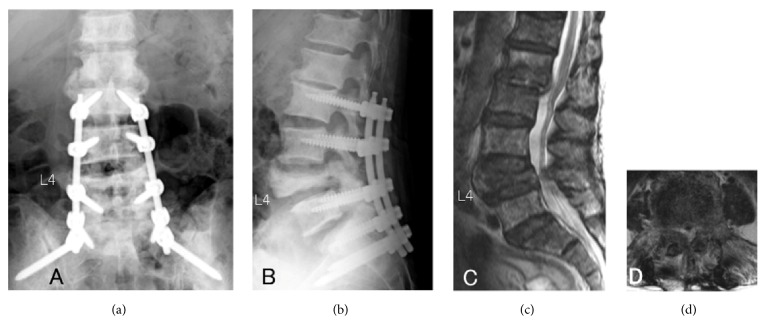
26 months after minimally invasive spine stabilization. (a) Posteroanterior view on radiography. (b) Lateral view on radiography. (c) Sagittal view on T2-weighted MRI. (d) Axial view of the L4 lumbar vertebrae on T2-weighted MRI.
